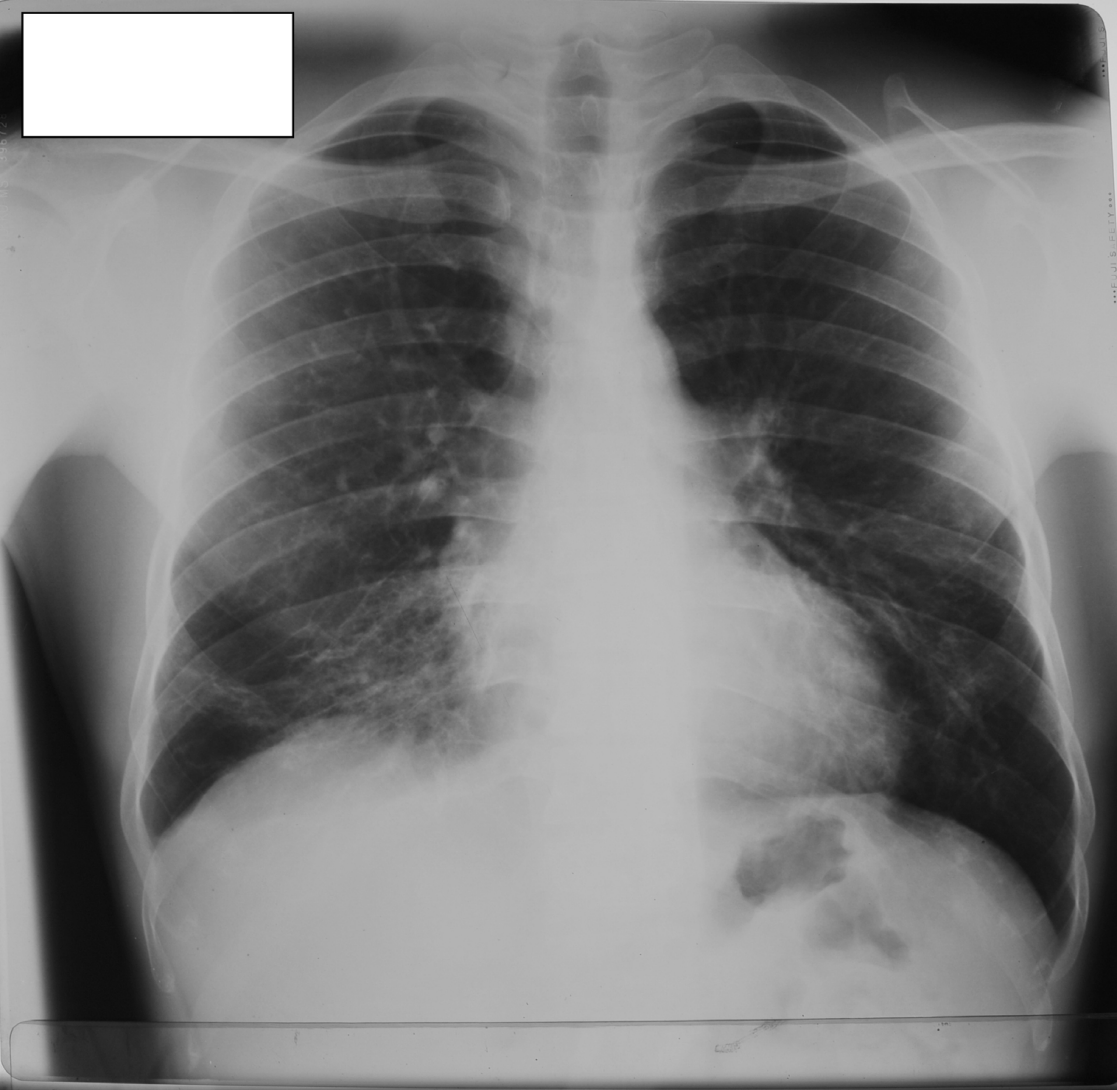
Chest roentgenogram from our patient. Features of previous pulmonary tuberculosis can be seen.
